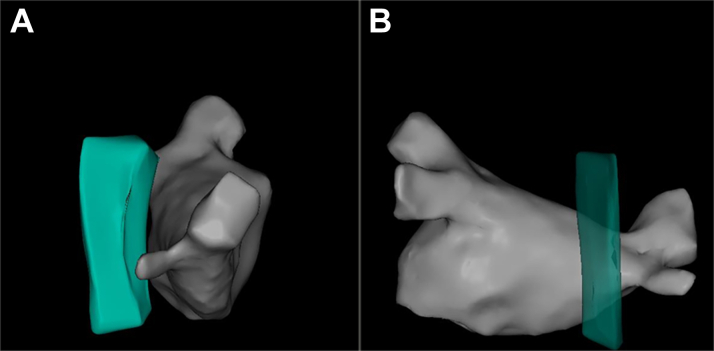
Relative Position Between the Pulmonary Veins and the Gastric Conduit Intracardiac echocardiography–based 3-dimensional reconstruction model shows the relative position between the pulmonary veins and the gastric conduit: (A) right lateral view and (B) posteroanterior view.
